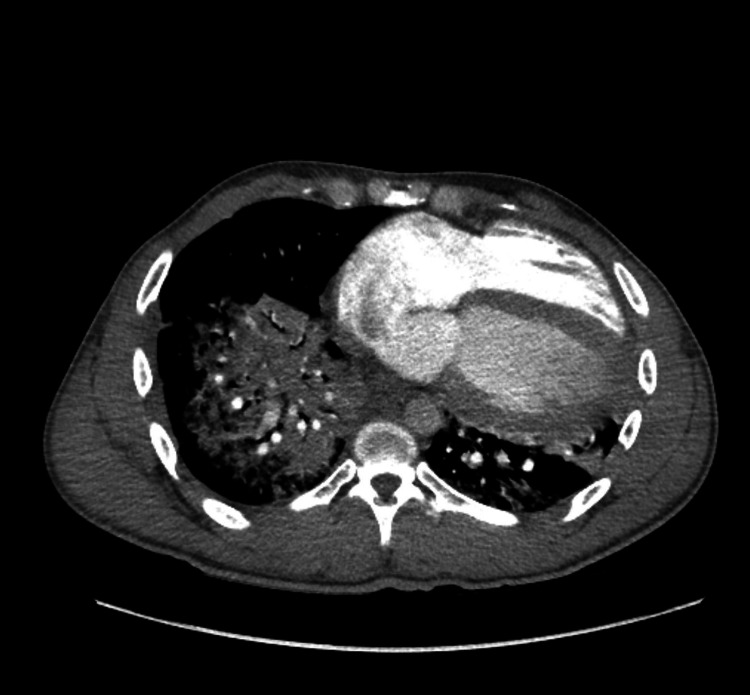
CT angiogram chest axial slice displaying a dense central alveolar pneumonia that extends in bilateral lung fields. CT, computed tomography
